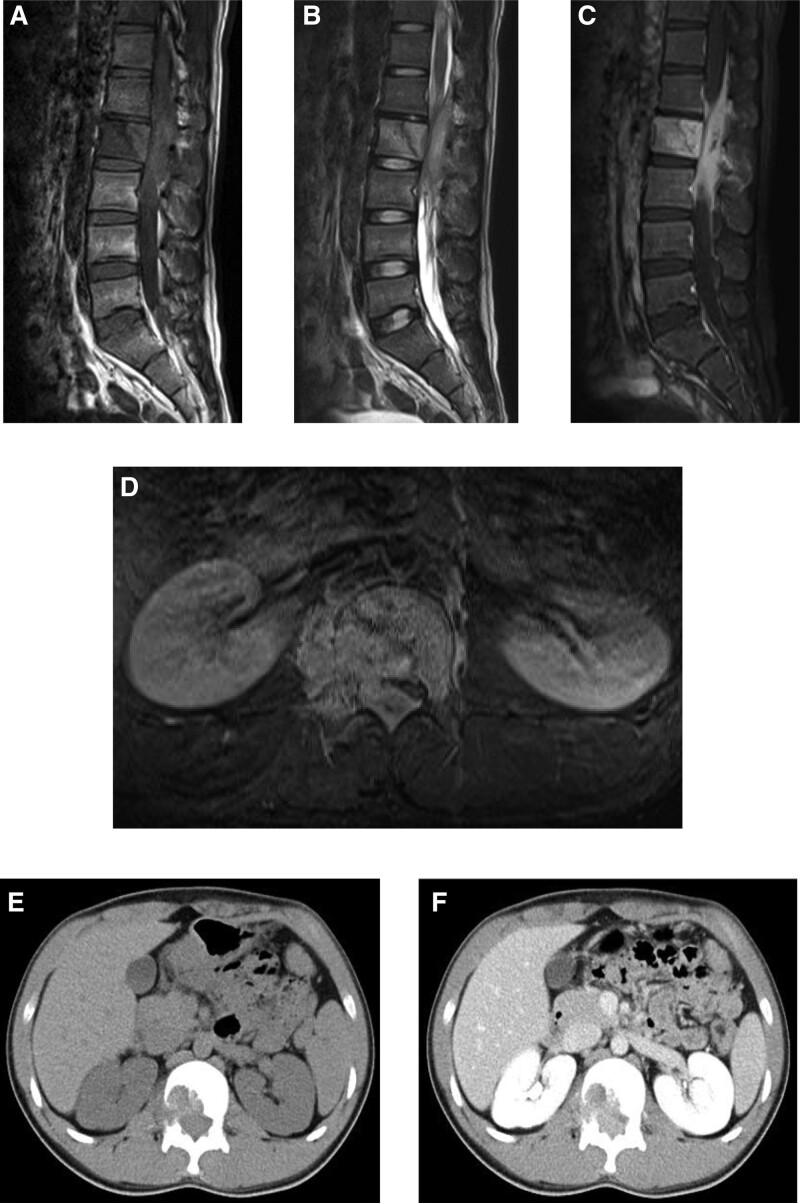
(A) Preoperative sagittal T1-weighted magnetic resonance (MR) image showing that the L2 vertebral body and contiguous epidural space-occupying lesion are of moderately heterogeneous iso signal intensity. (B) Preoperative sagittal T2-weighted MR images showing that the L2 vertebral body and contiguous epidural space-occupying lesion have moderately heterogeneous high signal intensity. (C) Preoperative sagittal gadolinium-enhanced fat-suppressed MR image showing that the L2 vertebral body and contiguous epidural space-occupying lesion have a high signal intensity. (D) Preoperative axial gadolinium-enhanced fat-suppressed MR axial image demonstrating that the lesion extended from the L2 vertebral body to the right extravertebral and epidural spaces, severely compressing the dural tube from the right side. (E) Preoperative axial computed tomography (CT) image showing an osteolytic lesion on the right posterior side of the L2 vertebral body. (F) Preoperative axial CT with contrast enhancement showing that the space-occupying epidural lesion was moderately enhanced.
